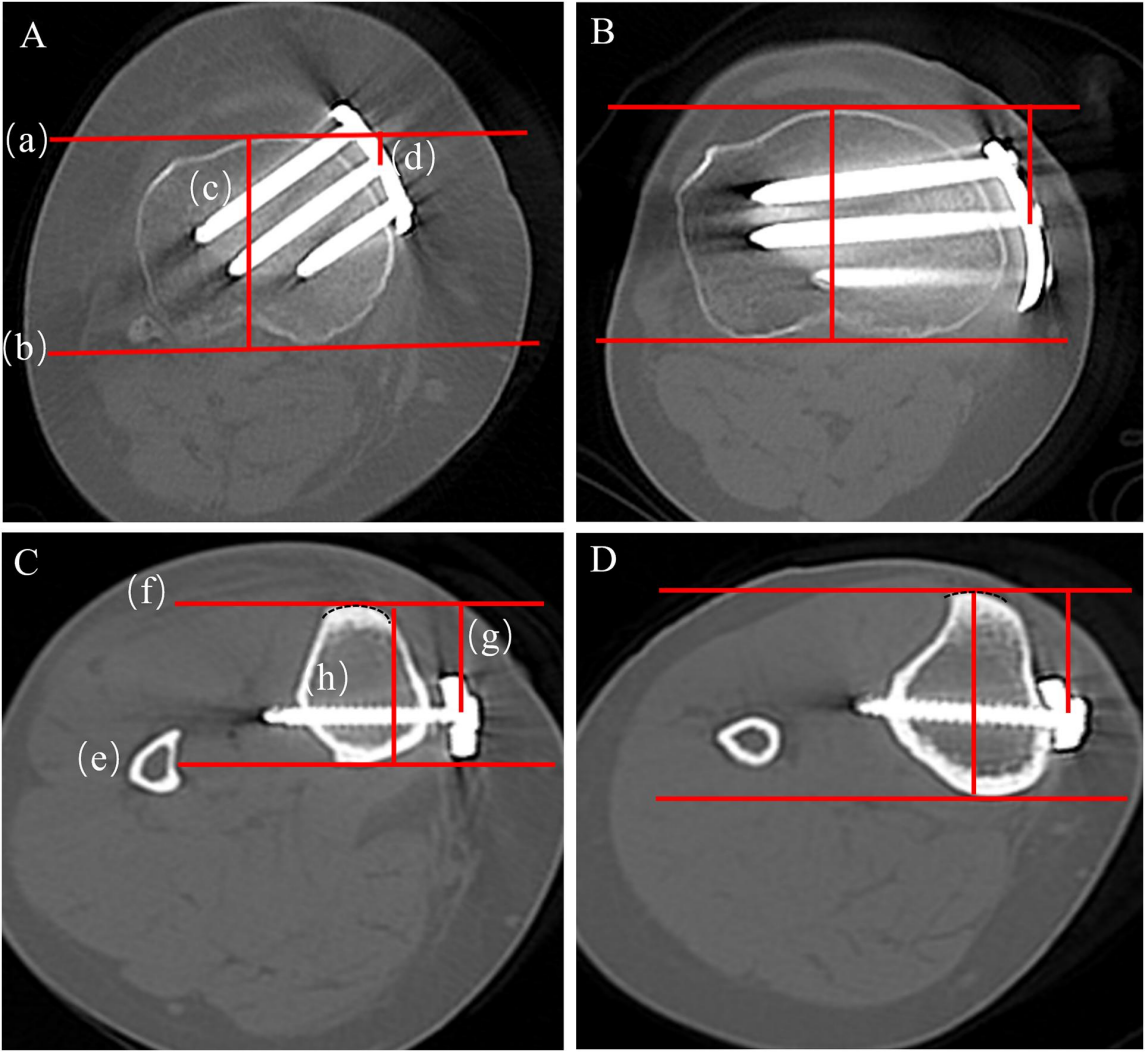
Axial CT measurement of proximal and distal plate position. (A) Proximal position-T-shaped locking plate; (B) proximal position novel locking plate; (C) distal position-T-shaped locking plate; (D) distal position novel locking plate.
